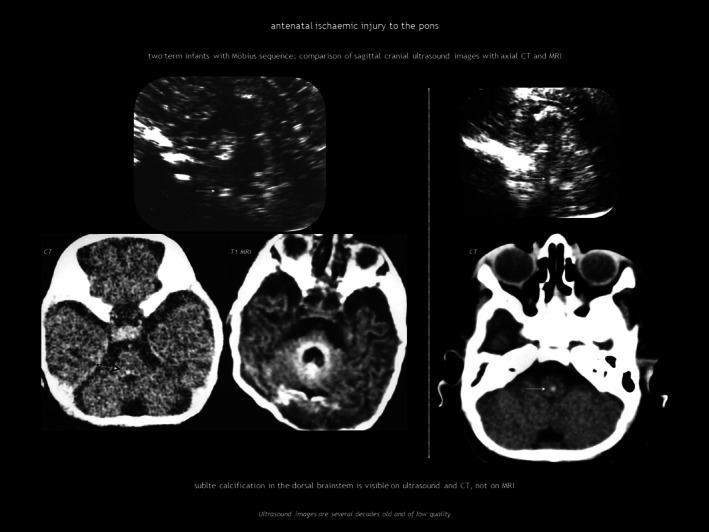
Antenatal ischaemic injury to the pons. Abbreviations: CT, computed tomography; MRI magnetic resonance imaging.
